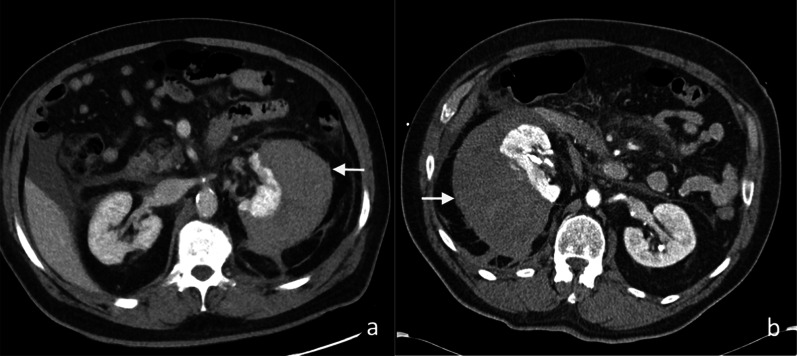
Axial contrast-enhanced CT images of (a) perinephric haematoma (white arrow), and (b) subcapsular haematoma (white arrow) post-ESWL in two patients
